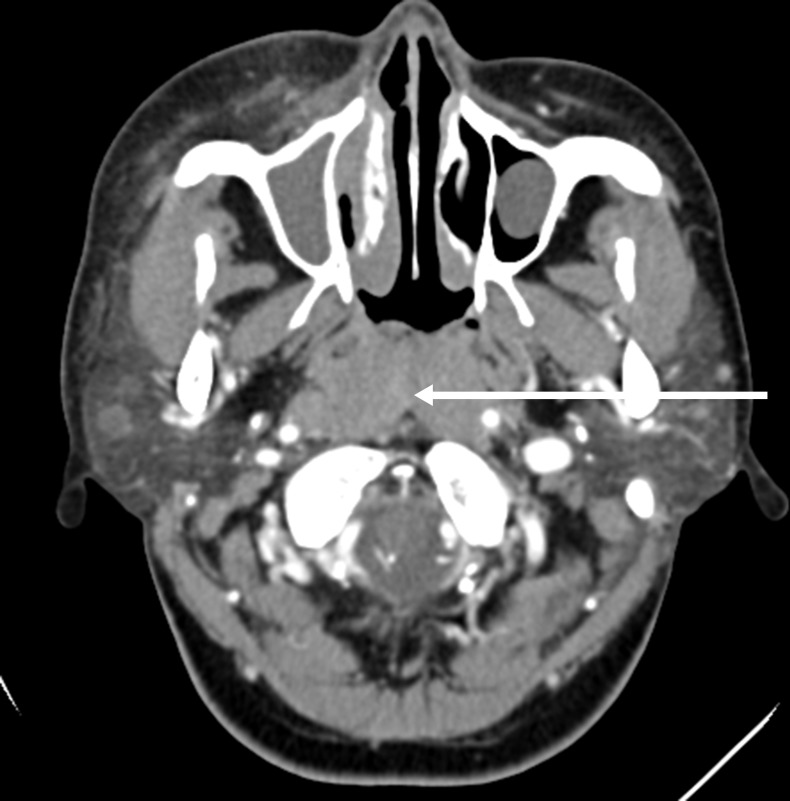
Contrast brain computed tomography shows diffuse swelling of the posterior wall of the pharynx with mild enhancement
